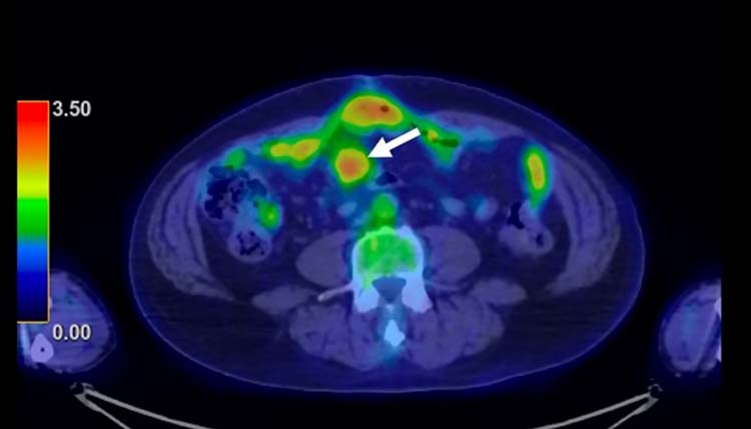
PET-CT finding. PET-CT showed that the maximum standardized uptake value (SUV max) of the mesenteric tumor was 2.9, with no other tumors present in the whole body.
